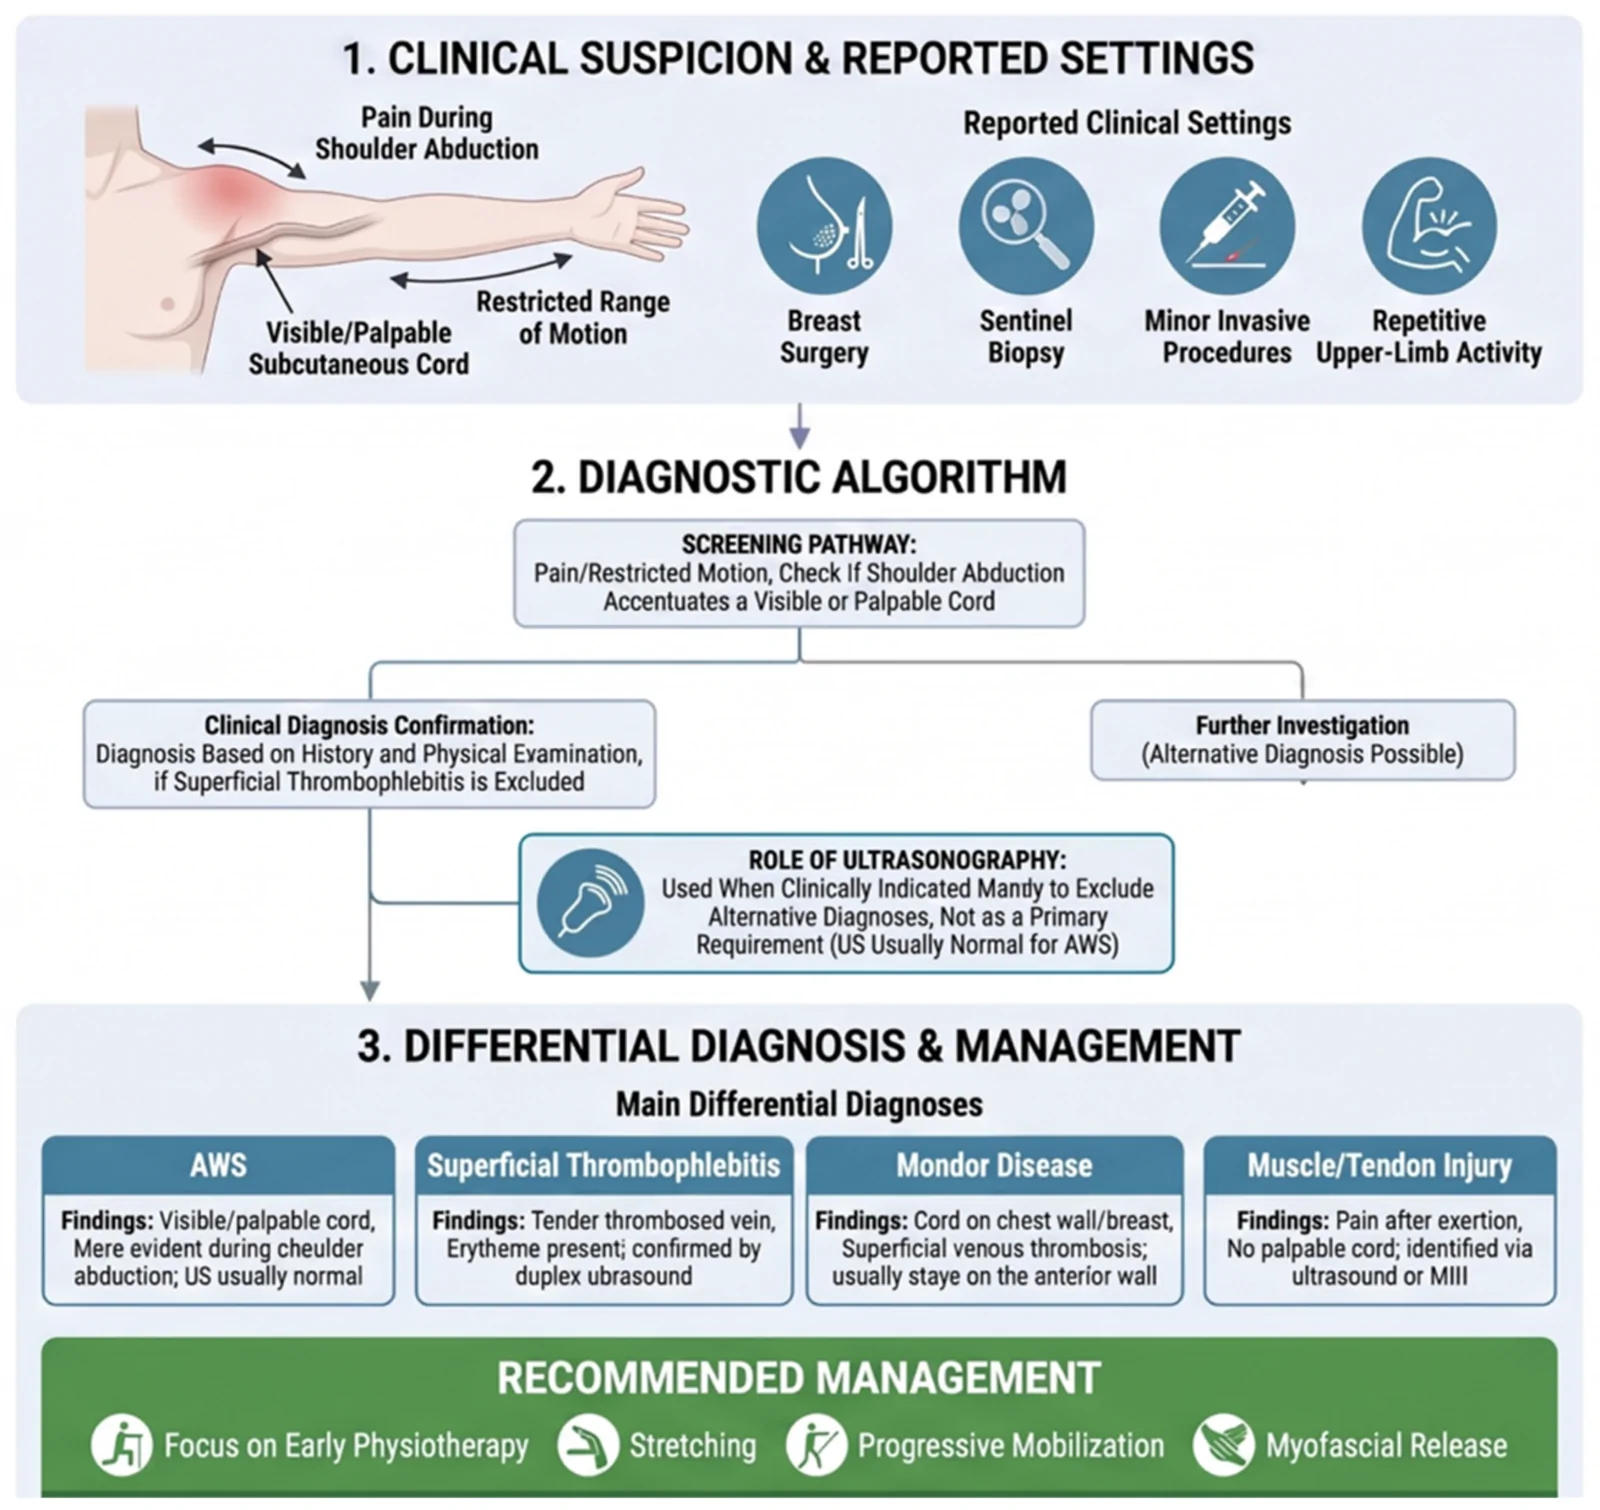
Diagnostic algorithm and clinical management of Axillary Web Syndrome (AWS). Proposed clinical approach for the diagnosis, differential diagnosis, use of ultrasonography, and conservative management of AWS.
